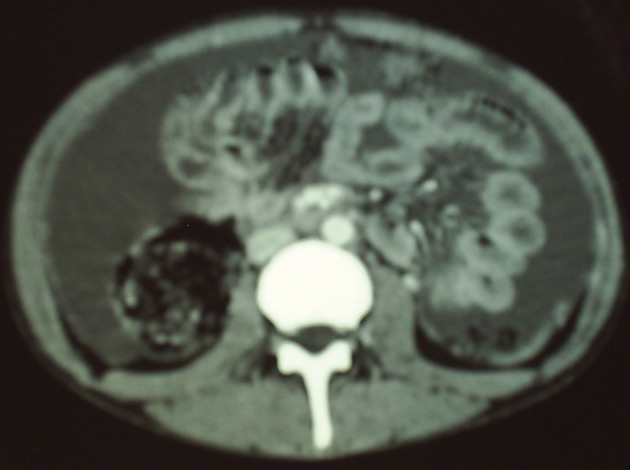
“Omental cake” apperance on abdominal CT.
